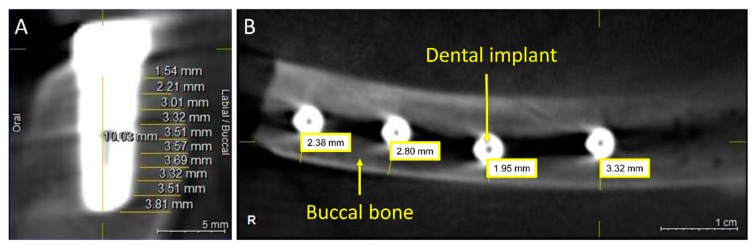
CBCT image of a dental implant and measurement points in the sagittal (A) and horizontal (B) view.
